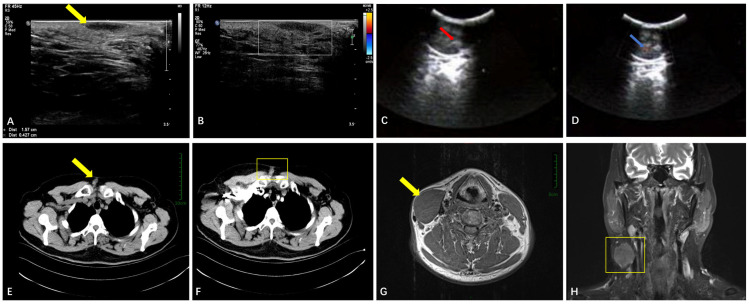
(A,B) A left perianal benign GCT, which was morphologically regular and smooth (see the yellow arrow) on ultrasound images and in which there was no colored blood flow signal; (C,D) a malignant GCT in the back with a regular morphology, clear borders, uneven internal echo (see the red arrow), and a colored blood flow signal on ultrasound images (blue arrow); (E,F) a CT scan and an enhanced image of a right anterior GCT in the chest wall showing a uniform density of nodular shadows (yellow arrow) and the enhanced scan showing enhancements (yellow border); (G,H) MRI images of a GCT in the right sternocleidomastoid muscle showed an equally weighted signal on T1WI (yellow arrow) and an uneven hyperintense shadow on T2WI (yellow border). (E,F) share a bar, (G,H) share a bar.
